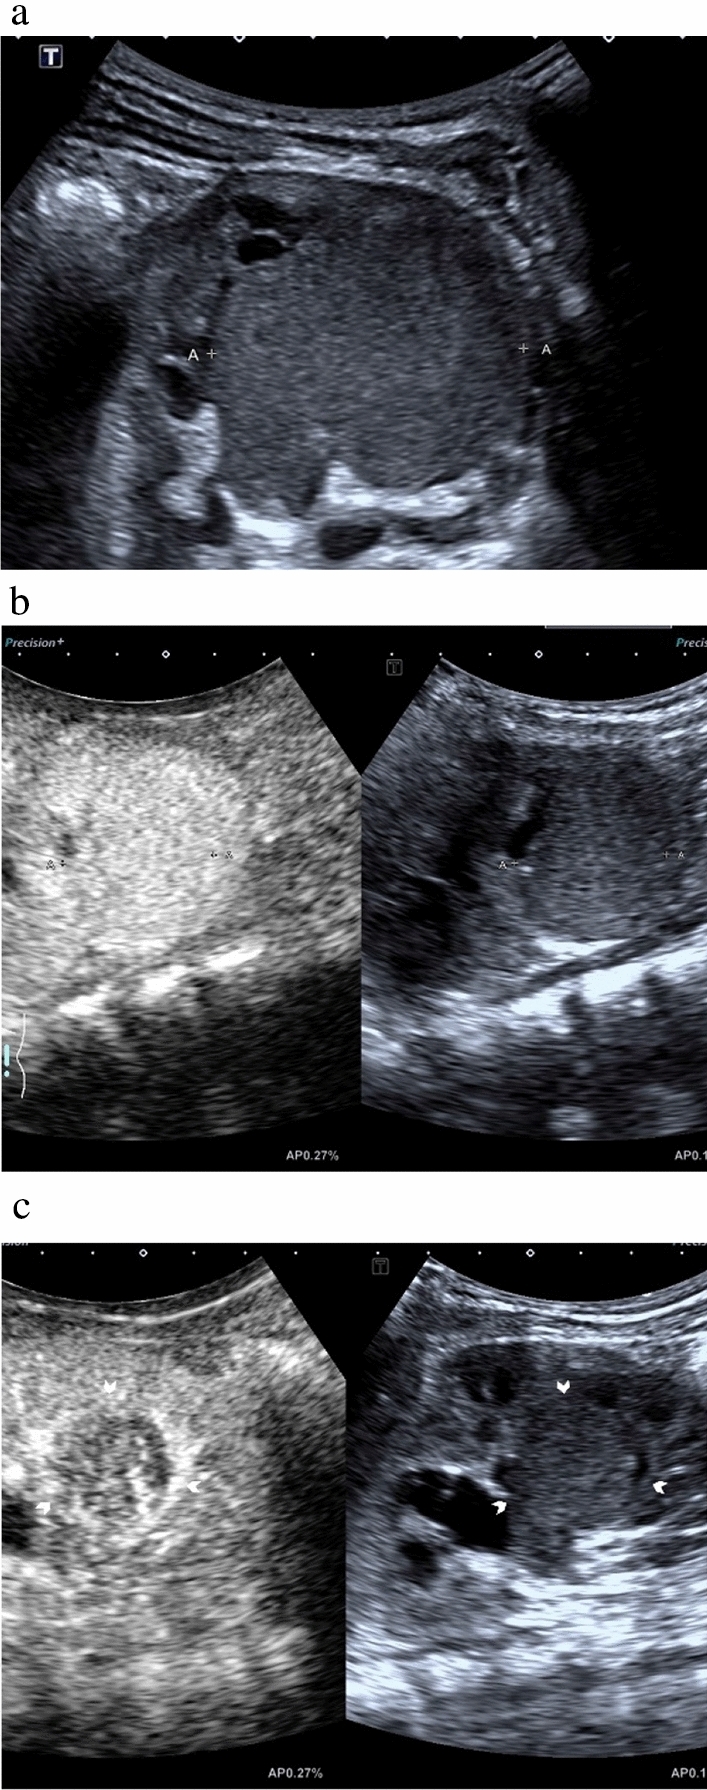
Images in a 2-year and 7-month-old boy with confirmed Wilms tumor. (a) Gray-scale ultrasound shows a solitary 5.5-cm homogeneous isoechoic lesion (caliber) in the left kidney. Contrast-enhanced ultrasound images of the lesion demonstrate (b) homogeneous isoenhancement in cortical phase (19 s) (caliber) and (c) wash-out in parenchymal phase (45 s) (arrowheads).
